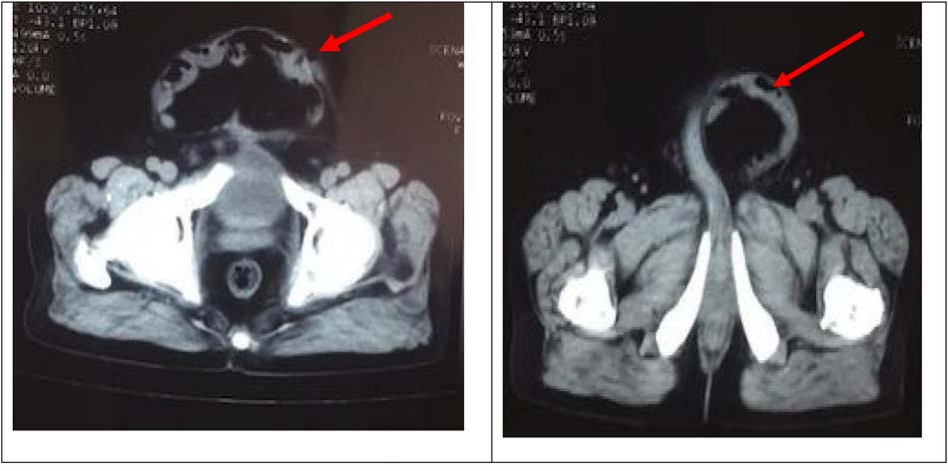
CT of the abdomen confirmed thinning of the rectus sheath with focal outpouching of rectus in the infra umbilical region and herniation of small bowel loops. Red arrows showing herniation of small bowel in infraumbilical region.
